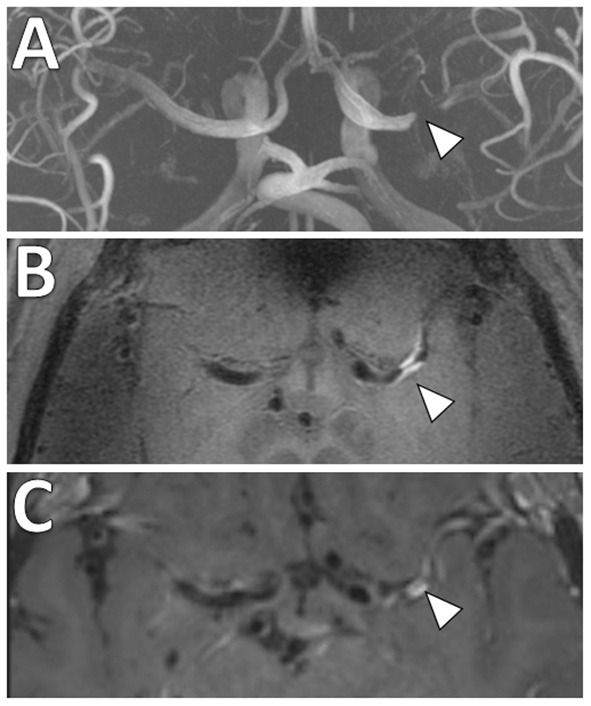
Comparison of 3 and 7 Tesla (T) VW-MRI for intracranial atherosclerosis. A patient with history of obesity, hyperlipidemia and hypertension and ischemic stroke underwent a (A) 7T TOF-MRA, which showed left M1 middle cerebral artery severe stenosis (arrowhead). (B) 7T MRI axial T1 SPACE post-gadolinium (0.5 mm isotropic resolution) image clearly delineates arterial wall thickening and avid vessel wall enhancement in the region of stenosis (arrowhead). (C) The same patient was imaged 18 days later on a 3T MRI (T1 SPACE post-gadolinium, 0.8 × 0.8 × 1.0 mm) to assess the stenosis (arrowhead). The 7T VW-MRI shows improved conspicuity and delineation of the margins of the arterial wall compared to the 3T, an advantage of the higher magnet strength due to the higher signal to noise leveraged for higher spatial resolution and soft tissue contrast.
